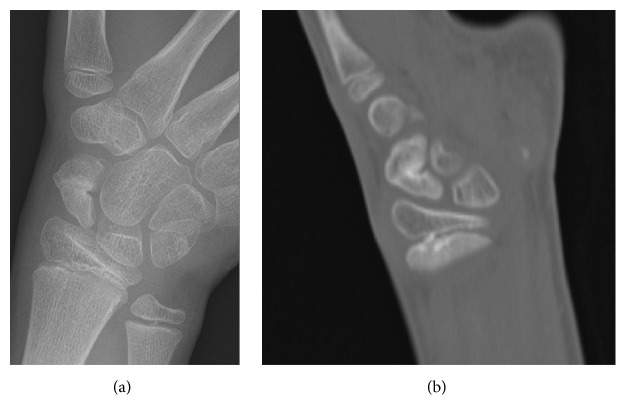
(a) Plain radiograph at the end of cast immobilization showing improved consolidation. (b) CT scan be performed one month after the end of cast immobilization showing union of the fracture.
